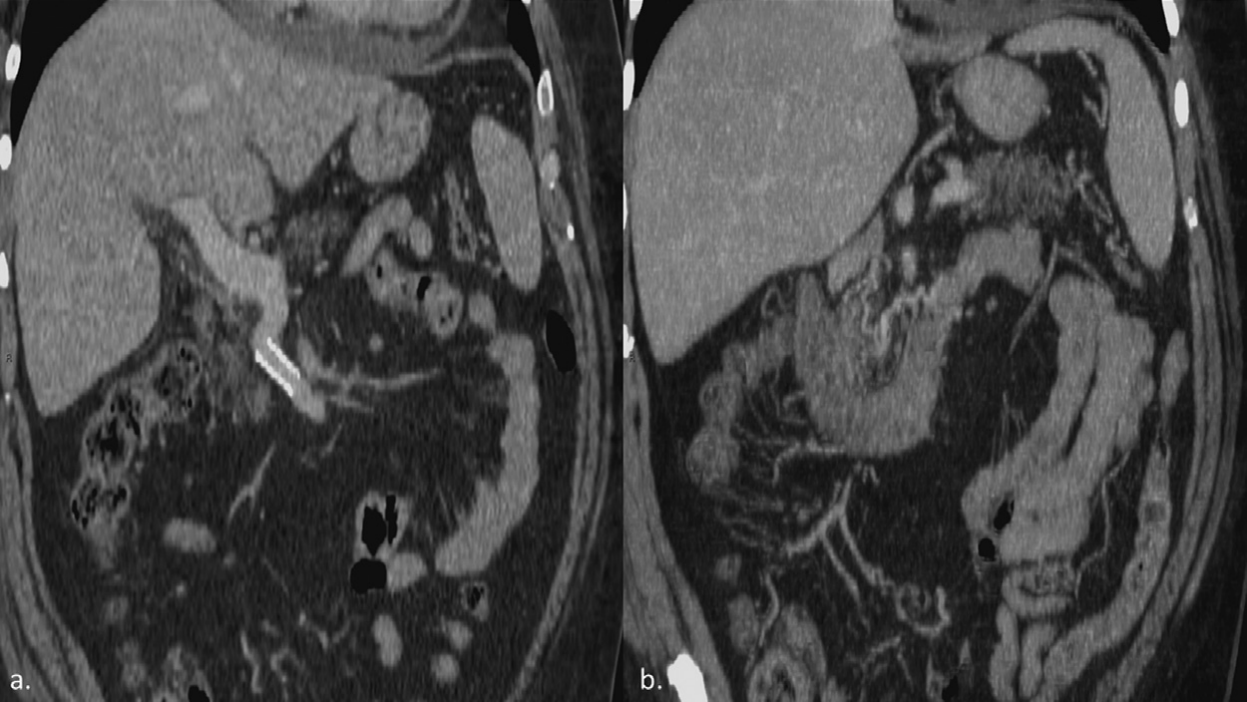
One month control CT-scan. Contrast-enhanced CT scan performed after one month showed SMV stent patency (a) and resolution of jejunal collateral varicosities (b).
